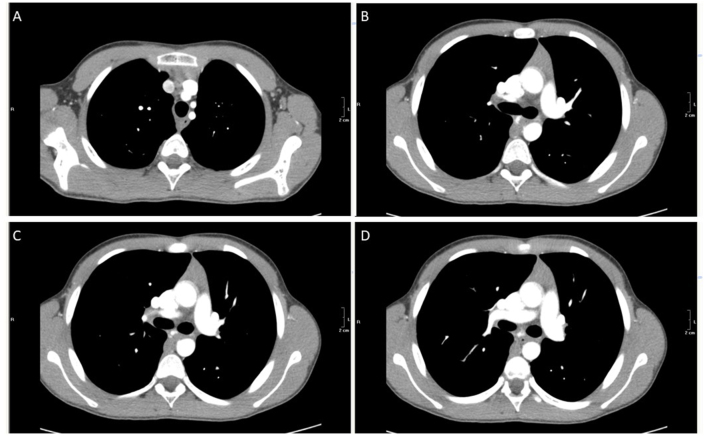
CT scan showing an area of abnormal contrast enhancement extending from the carina to the main bronchus, consistent with an angioma.
